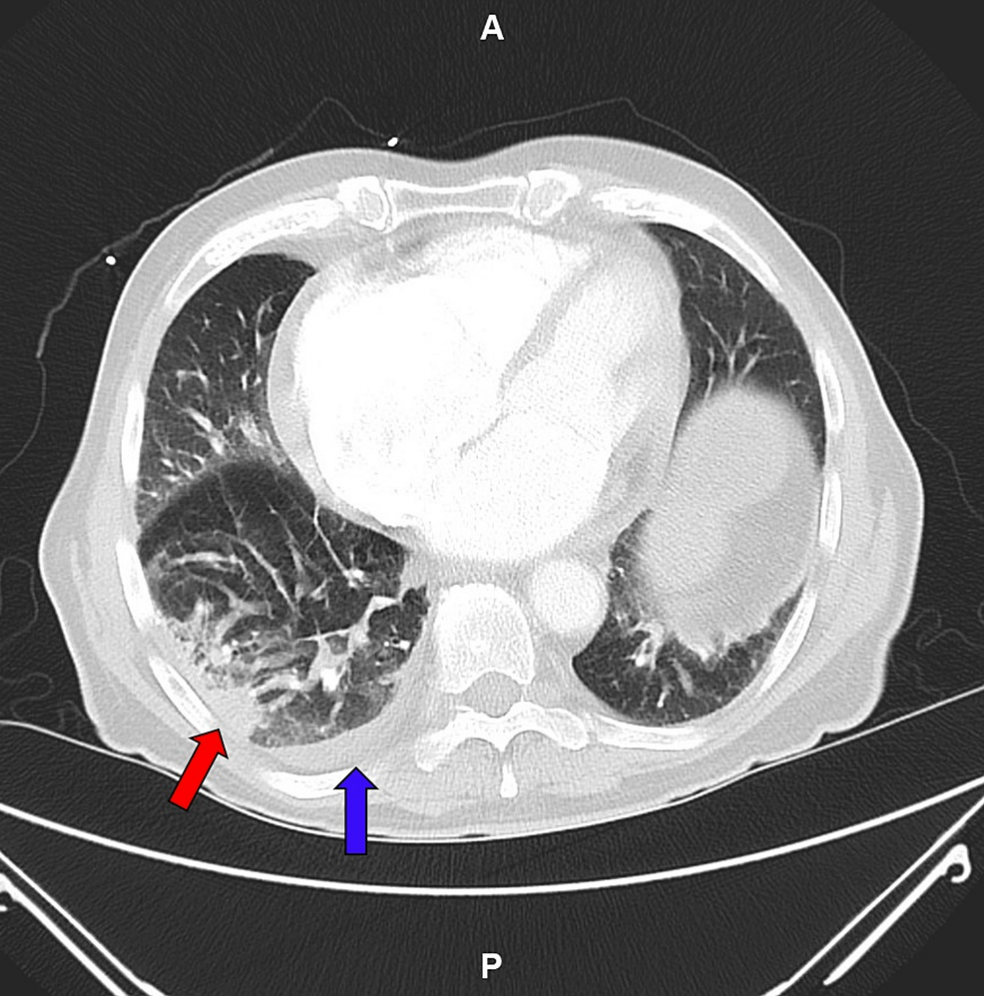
CTA pulmonary, axial view, showing a right-sided lower lung lobe consolidation (red arrow) and pleural effusion (blue), both consistent with pneumonia At this point in the hospital stay, further invasive investigation of the pleural effusion with thoracentesis was not pursued as the etiology of the fluid was presumed to be infectious. CTA: computed tomography angiogram
